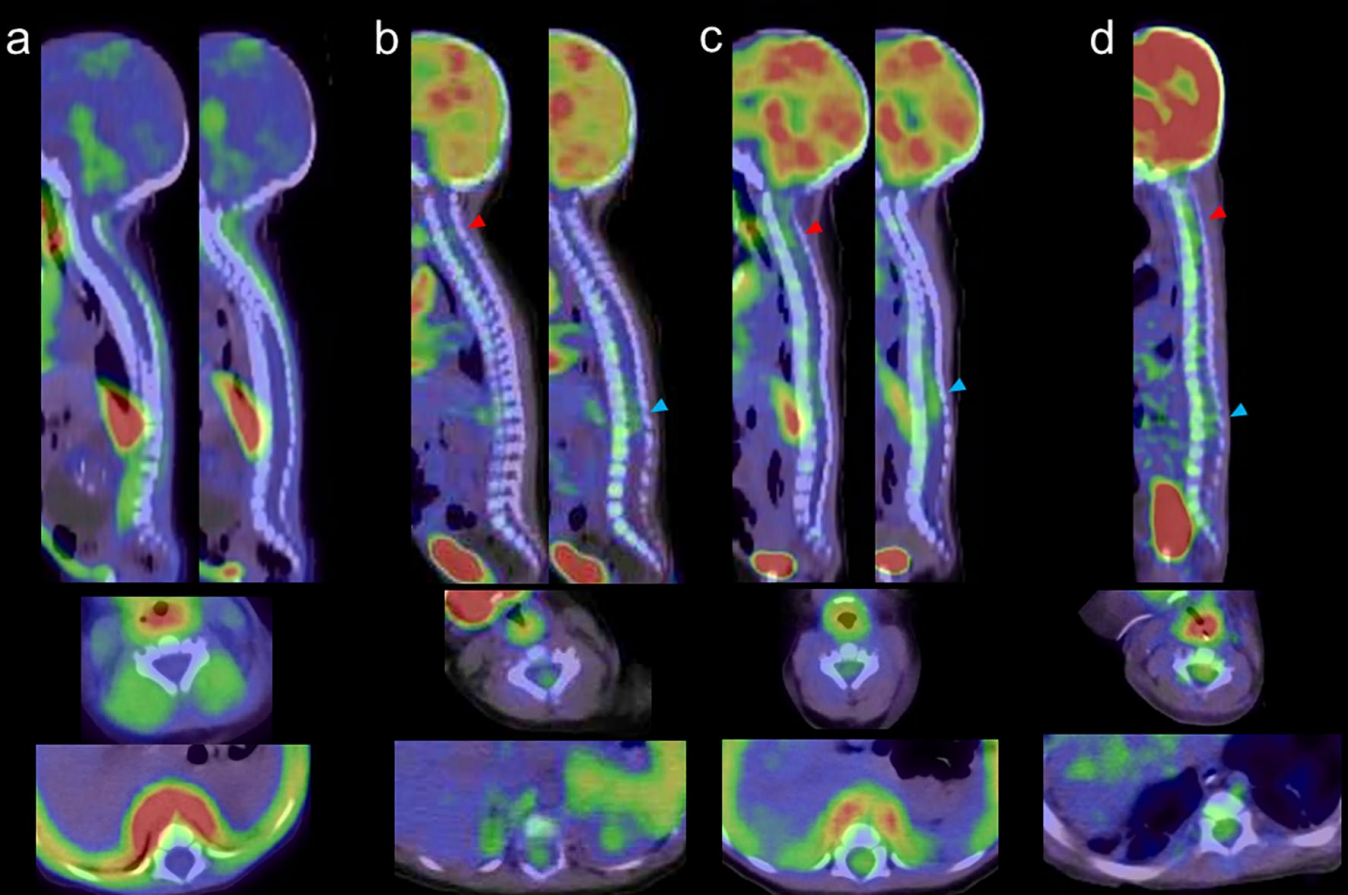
Sagittal and axial FDG-PET images in neonates and infants, a 15 days after birth, b 38 days after birth, c 61 days after birth, d 107 days after birth. FDG uptake in the cervical (red arrowhead) and lower thoracic spinal cord (blue arrowhead) is visible
